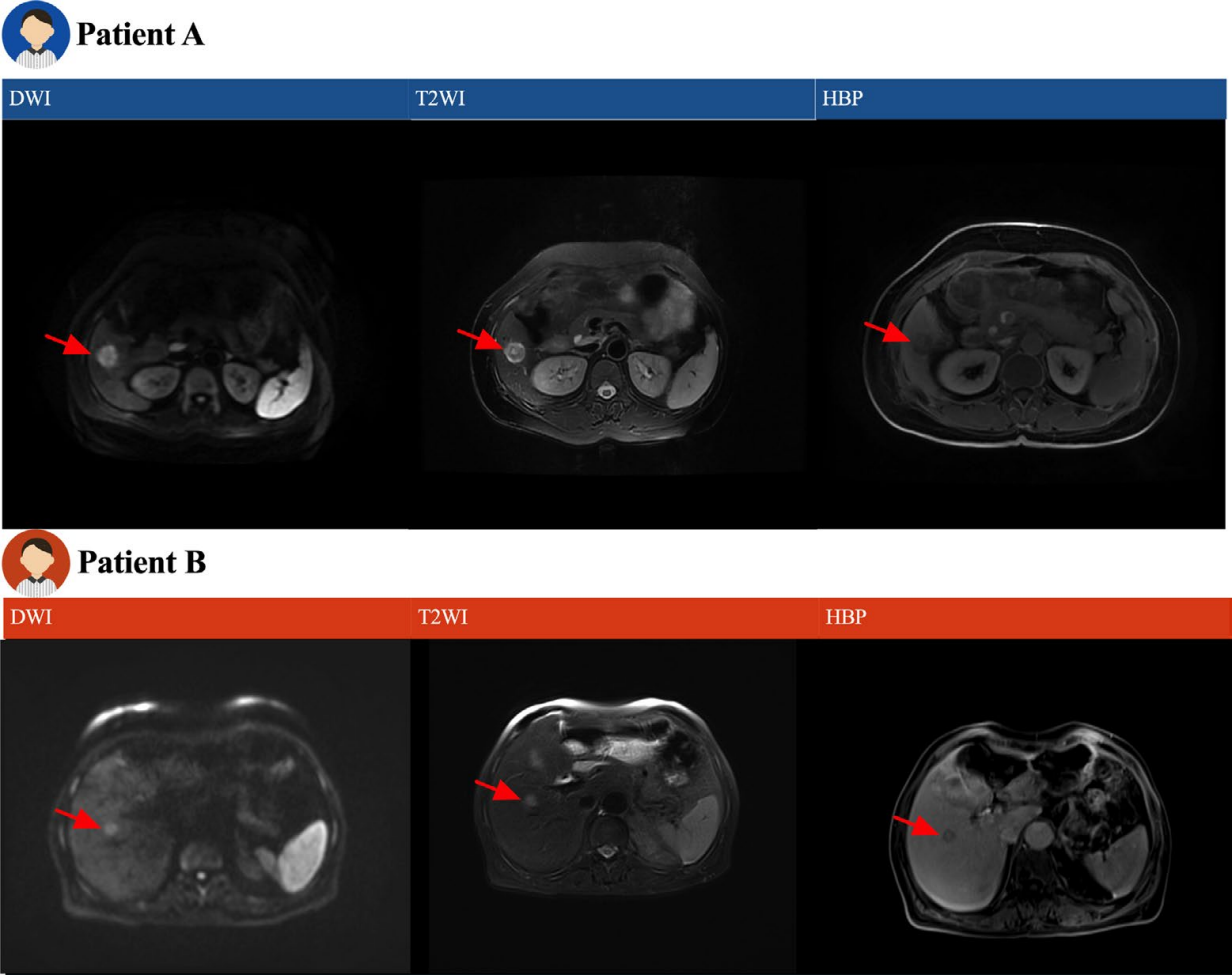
Representative cases of intrahepatic mass‐forming cholangiocarcinoma (IMCC) and colorectal liver metastasis (CRLM). Patient A: A 55‐year‐old male diagnosed with IMCC. Patient B: A 48‐year‐old male diagnosed with CRLM. DWI, diffusion‐weighted imaging; HBP, hepatobiliary phase; T2WI, T2‐weighted imaging.
